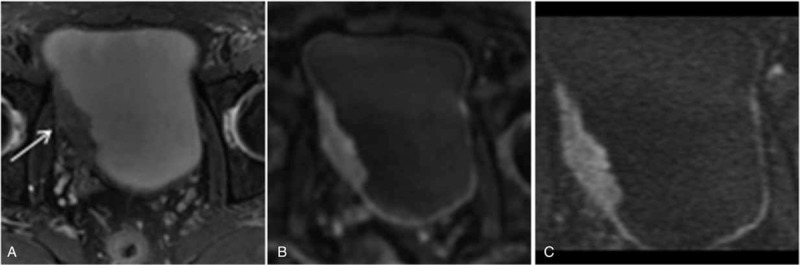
A 51-year-old man with stages of pT2b urothelial carcinoma. A, The axial T2-weighted MRI shows an ovoid mass along the right urinary bladder wall. The tumor extends into the perivesical fat with an irregular contour (arrow). B, The axial fFOV DW MRI shows a bulging tumor with a smooth margin on the right wall of the bladder. C, The axial rFOV DW MRI shows a bulging tumor with a smooth margin on right wall of the bladder more clearly. DW = diffusion-weighted, fFOV = full field of view, MRI = magnetic resonance imaging, rFOV = reduced field of view.
